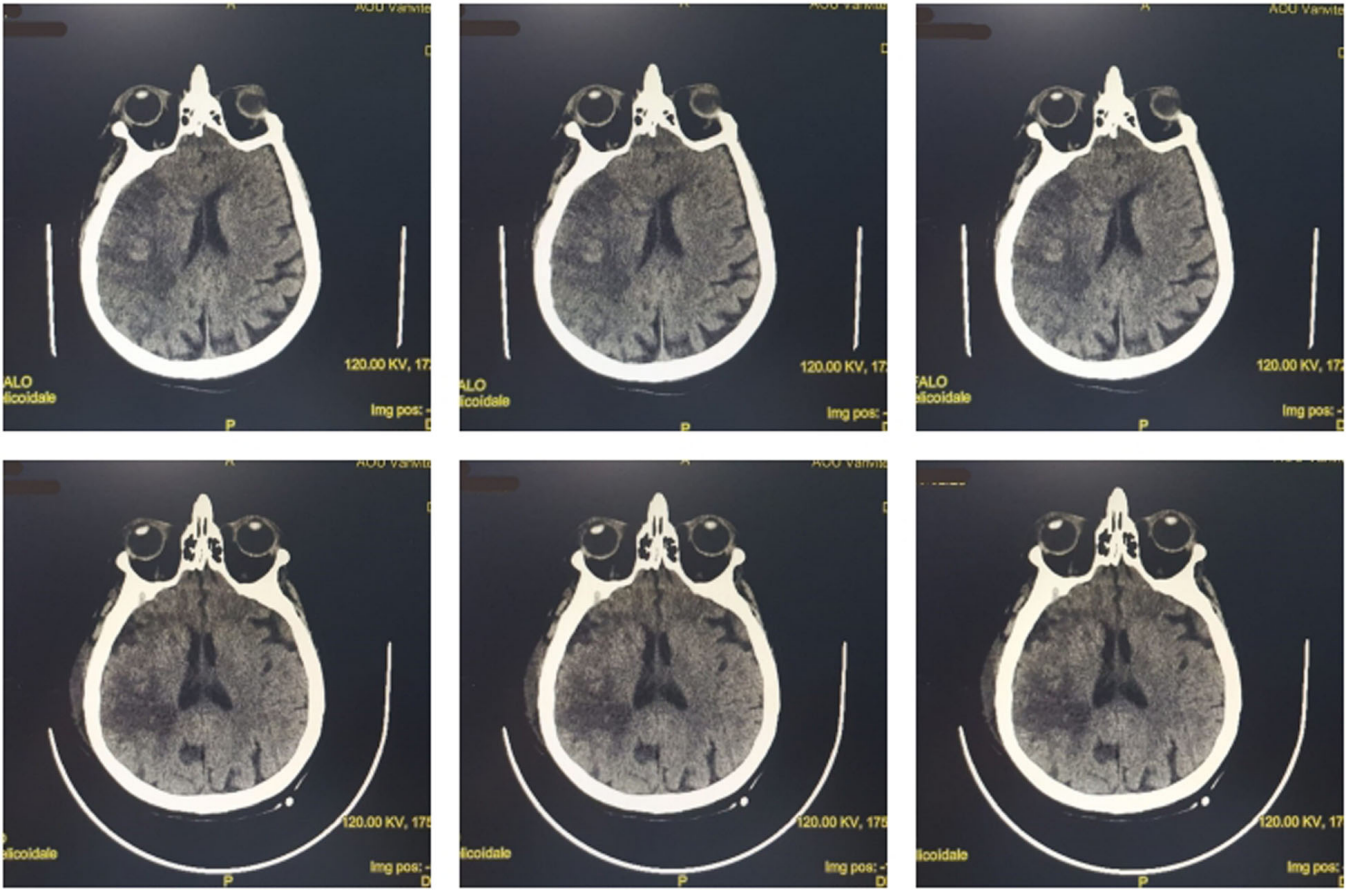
Brain computed tomography (CT). *Top row*: CT images upon arrival at the intensive care unit. *Bottom row*: CT images after 10 days of clinical evolution
